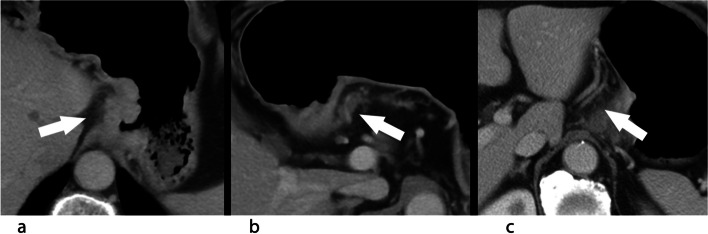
CT features of infiltrating peritumoral adipose space. a substantial density increase; b nodular intensities; c irregular linear opacities
